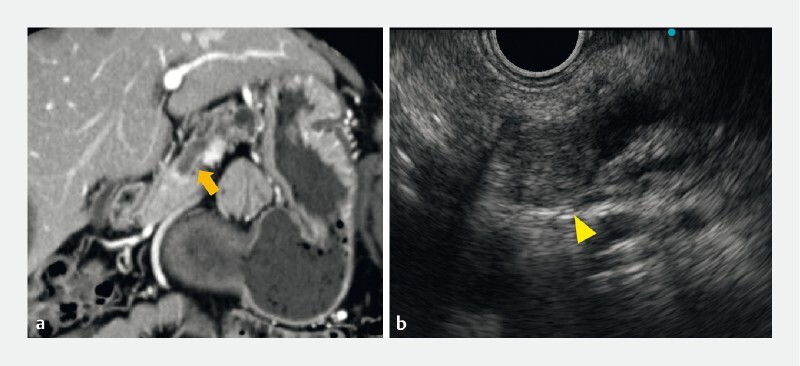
Computed tomography revealed an enhanced intraductal papillary tumor with main pancreatic duct dilation from the pancreatic body to the tail (arrow).
